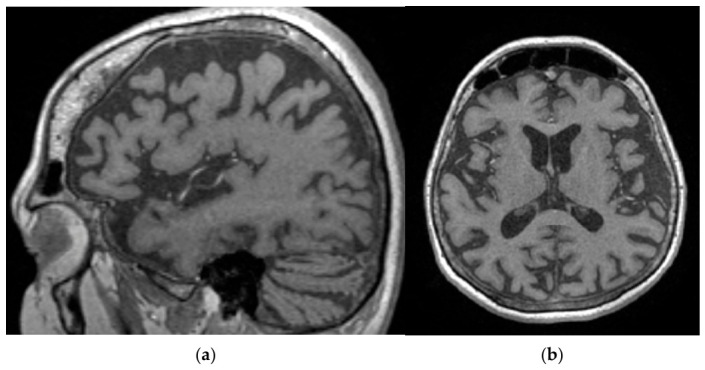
MRI scan: (a) and (b) bilateral frontotemporal volume loss with secondary ventriculomegaly.
